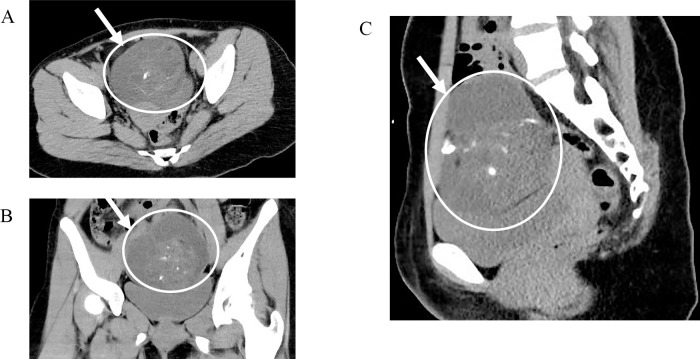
Abdominal plain CT scan. A: Cross-section, B: coronal section, and C: sagittal section. CT shows an intrapelvic tumor with internal calcification and fluid content containing fat (arrow).
